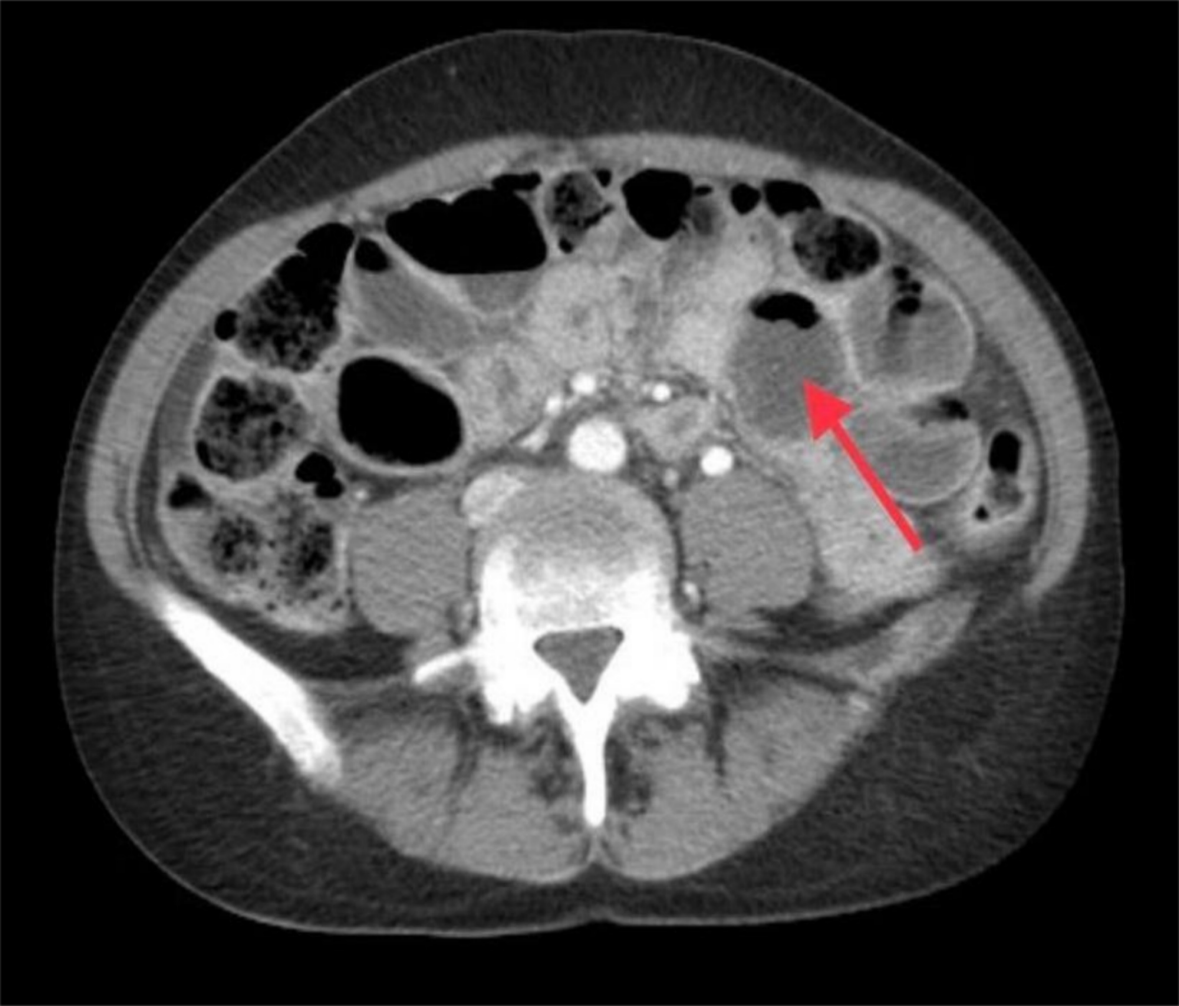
CT scan abdomen. Consistent with small bowel obstruction. Distended small bowel loop (Red arrow). CT: Computed tomography
